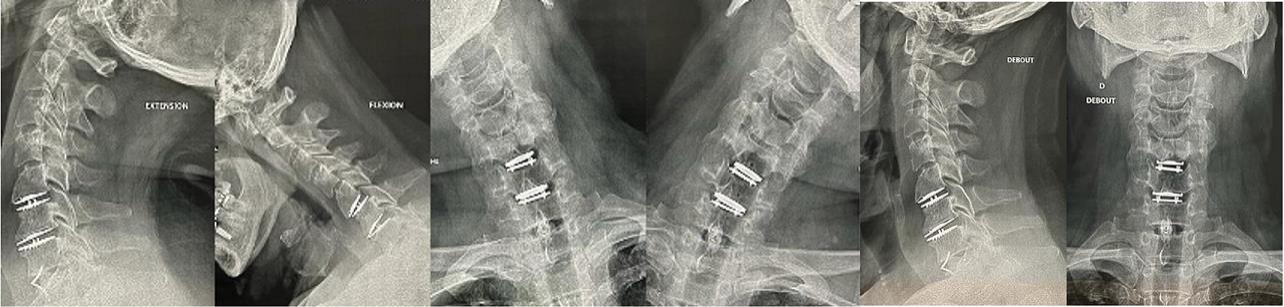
lateral (left) and frontal (right) static and dynamic X-ray of cervical spine showing the implants at 2 months
